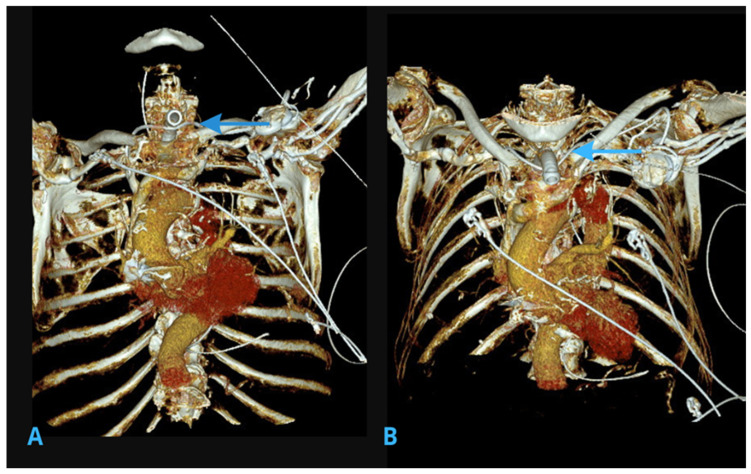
(Panel (A)) shows the inferior (bottom-up) view, while (panel (B)) presents the superior (top-down) perspective. A coronal computed tomography scan demonstrating the aberrant trajectory of the pacemaker lead through the inferior thyroid veins (highlighted by blue arrows), culminating in the lead fragment being positioned near the tracheostomy site.
